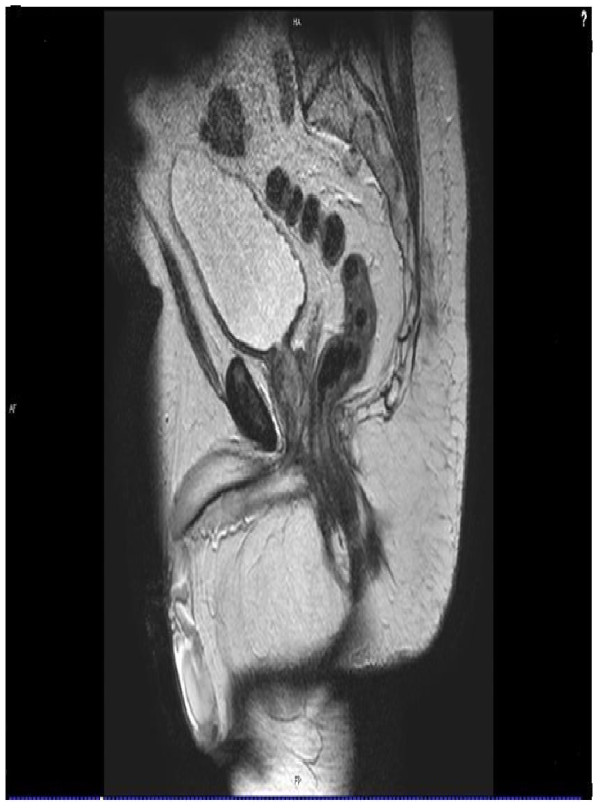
T2-weighted magnetic resonance image showing an anteverted coccyx and rectal impingement.
